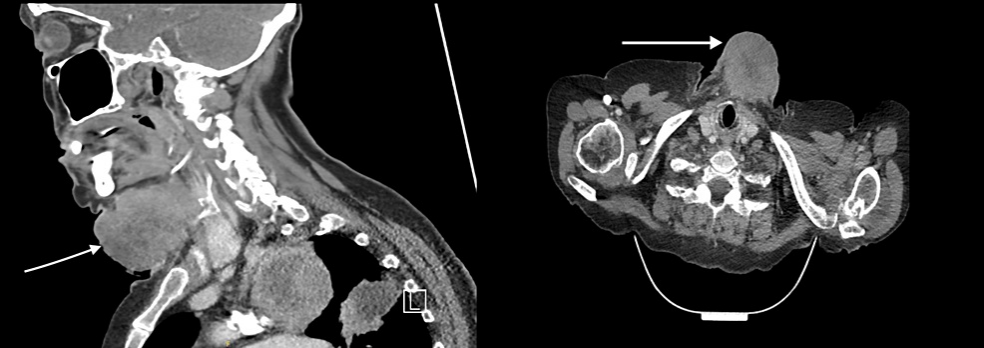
Computed tomography demonstrating large anterior neck subcutaneous deposit along with a lung primary (left: sagittal view, right: axial view)
